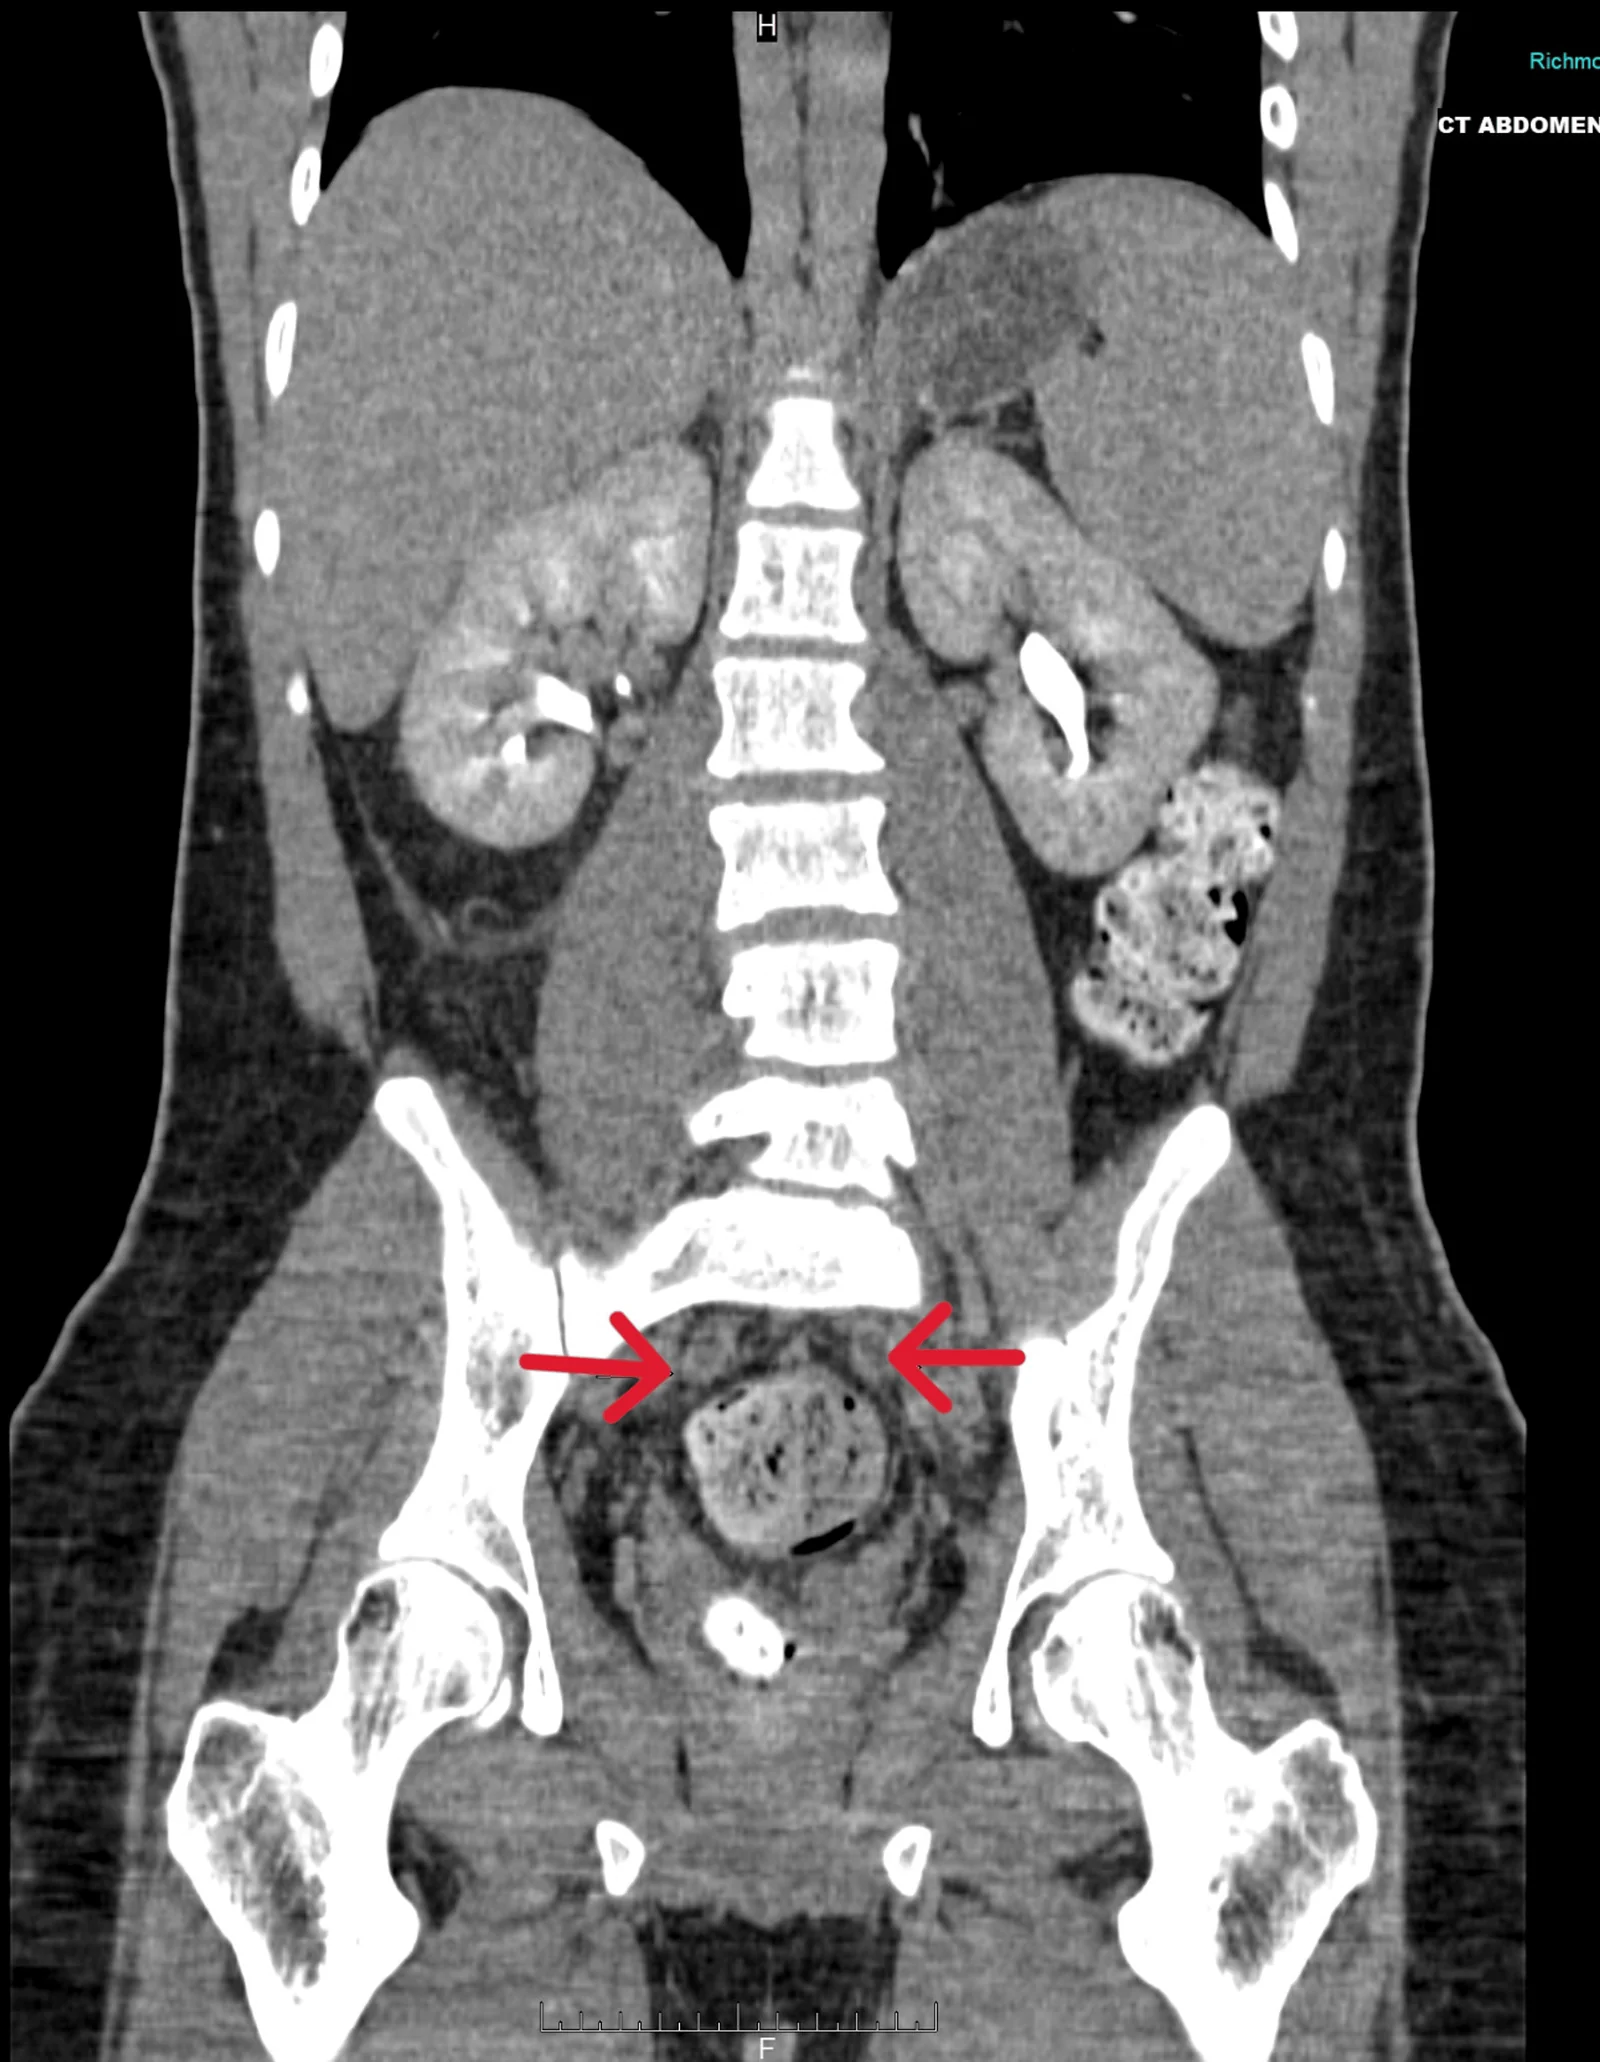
Initial coronal computed tomography angiography demonstrating extensive iliocaval thrombosis involving the pelvic venous system (red arrows)
